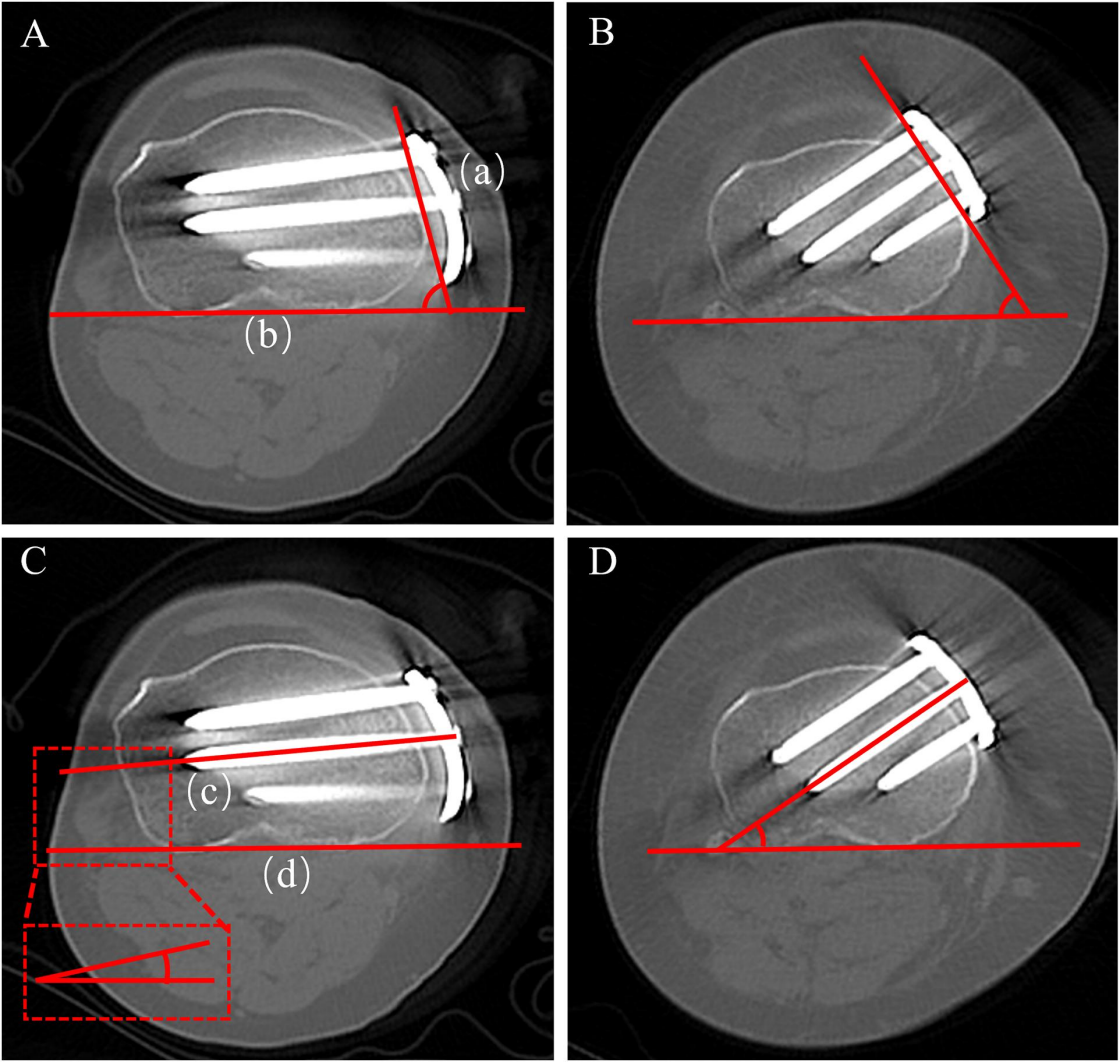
Measurement of plate angle and proximal central screw angle on axial CT. (A) Plate angle-novel locking plate; (B) plate angle-T-shaped locking plate; (C) proximal central screw angle-novel locking plate; (D) proximal central screw angle-T-shaped locking plate.
